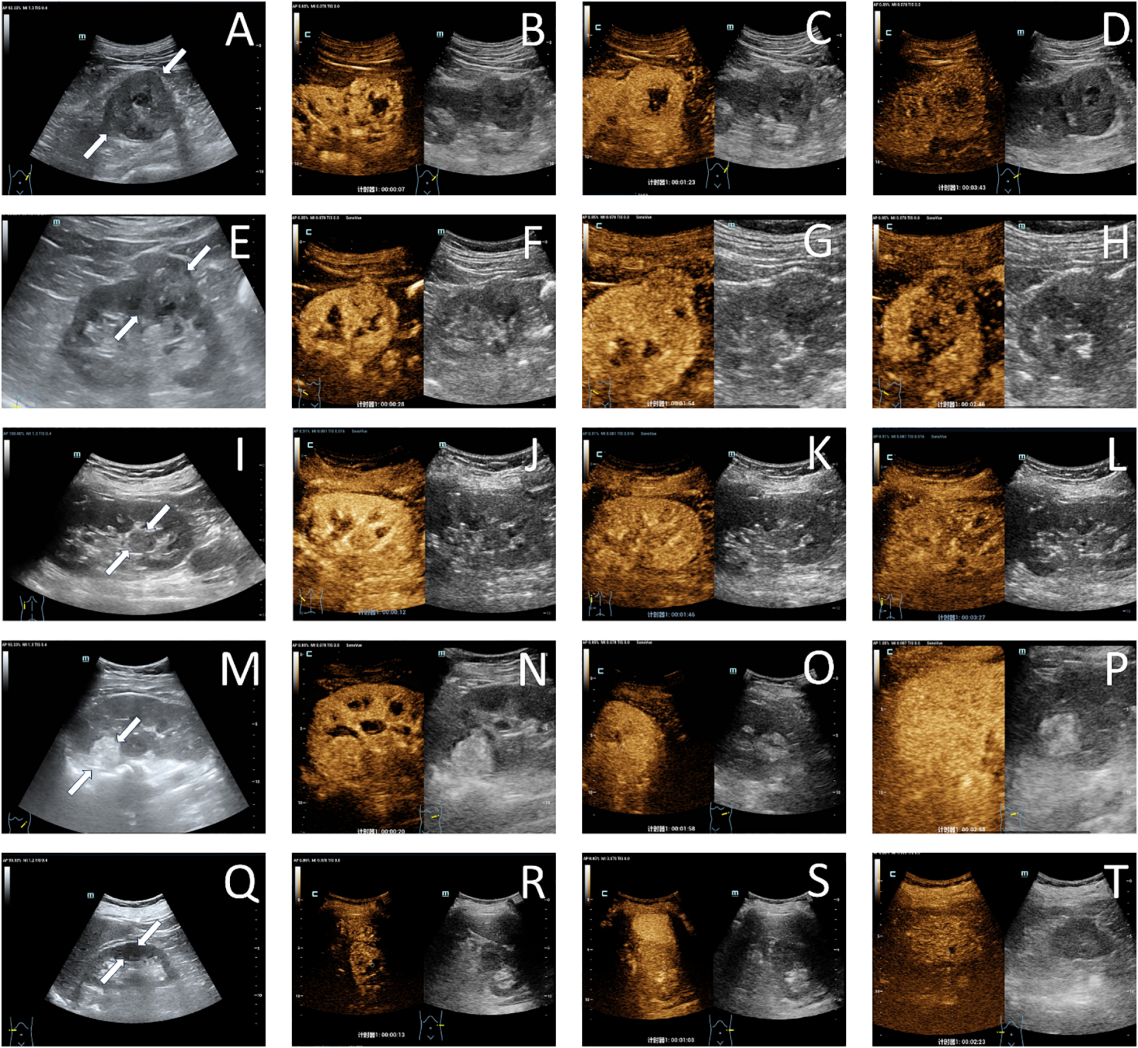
Ultrasonographic images of common renal tumors. A case of clear cell renal cell carcinoma in the left native kidney demonstrated a hypoechoic lesion (white arrow) on grayscale ultrasound imaging (A). During the cortical phase (B), the lesion exhibited heterogeneous hyperenhancement, followed by gradual washout in the medullary phase (C), and ultimately appeared as hypoenhancement in the delayed phase (D), with a non-enhancing liquefied necrotic area visible within the lesion; A case of papillary renal cell carcinoma in the right native kidney presented with a slightly hypoechoic lesion (white arrow) on grayscale ultrasound imaging (E). The lesion demonstrated mild hypoenhancement during the cortical phase (F) and subsequently underwent slow washout, exhibiting low enhancement in both the medullary phase (G) and the delayed phase (H); A case of chromophobe renal cell carcinoma in the left native kidney demonstrated a slightly hypoechoic lesion on grayscale ultrasound imaging, with a distinctive protrusion into the renal pelvis (white arrow) (I). The lesion exhibited uniformly mild hypoenhancement during the cortical phase (J), medullary phase (K), and delayed phase (L); A case of angiomyolipoma in the left native kidney presented with a slightly hyperechoic lesion (white arrow) on grayscale ultrasound imaging (M). The lesion demonstrated isoenhancement during the cortical phase (N), medullary phase (O), and delayed phase (P); A case of oncocytoma in the right native kidney demonstrated a hypoechoic lesion (white arrow) on grayscale ultrasound imaging (Q). The lesion exhibited synchronous enhancement during the cortical phase (R), medullary phase (S), and delayed phase (T), with a linear non-enhancing area visible in the center of the lesion.
